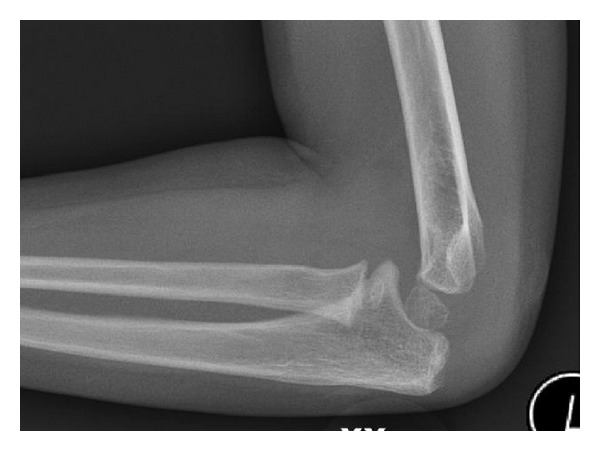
Lateral radiograph four months postoperatively. Note the union of the olecranon fracture and the satisfactory position of the radiocapitellar joint.
